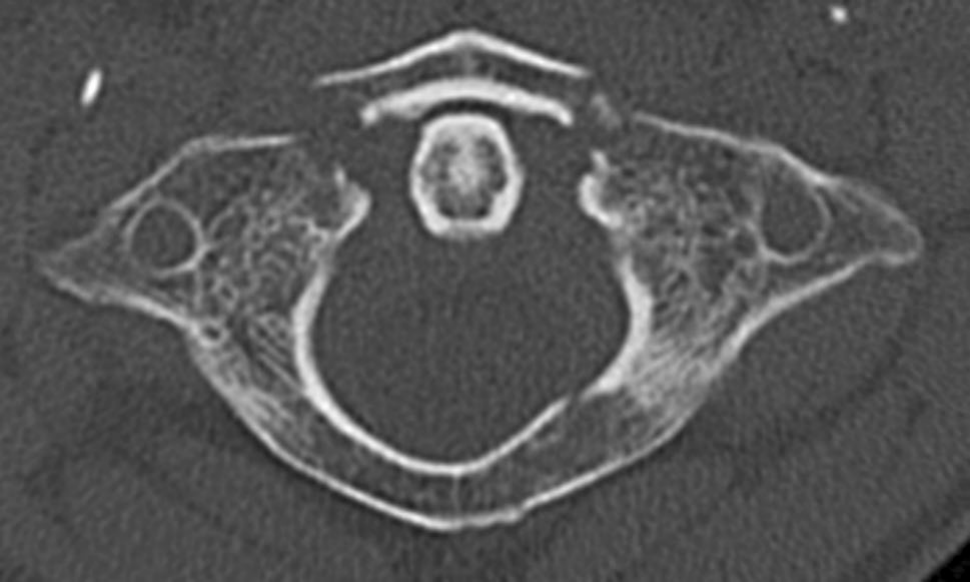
Section of a CT sequence in axial reconstruction of an exemplary case rated by the observers. This case was rated by all observers as a Gehweiler type 3 fracture, but the subclassification into type 3a (50%) and type 3b (50%) was inconsistent and all observers recommended additional magnetic resonance imaging. While four spine surgeons opted for a non-surgical treatment, one observer suggested a Goel–Harms fixation and one observer an isolated atlas osteosynthesis. *CT* computed tomography
